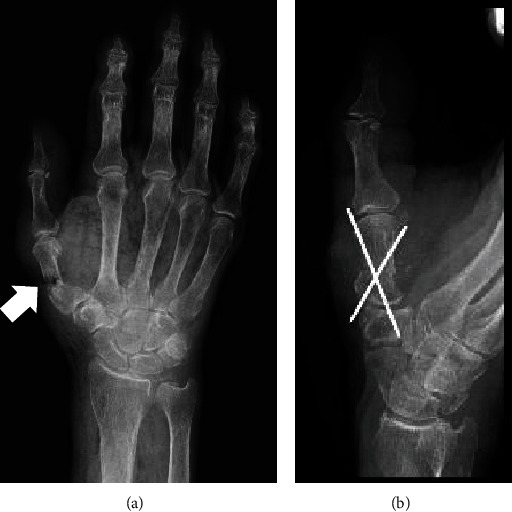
(a) The fracture in the first metacarpal shaft (arrow) is visible on the radiograph taken at the patient's first visit to our hospital. (b) The fracture was fixed by cross-pinning.
